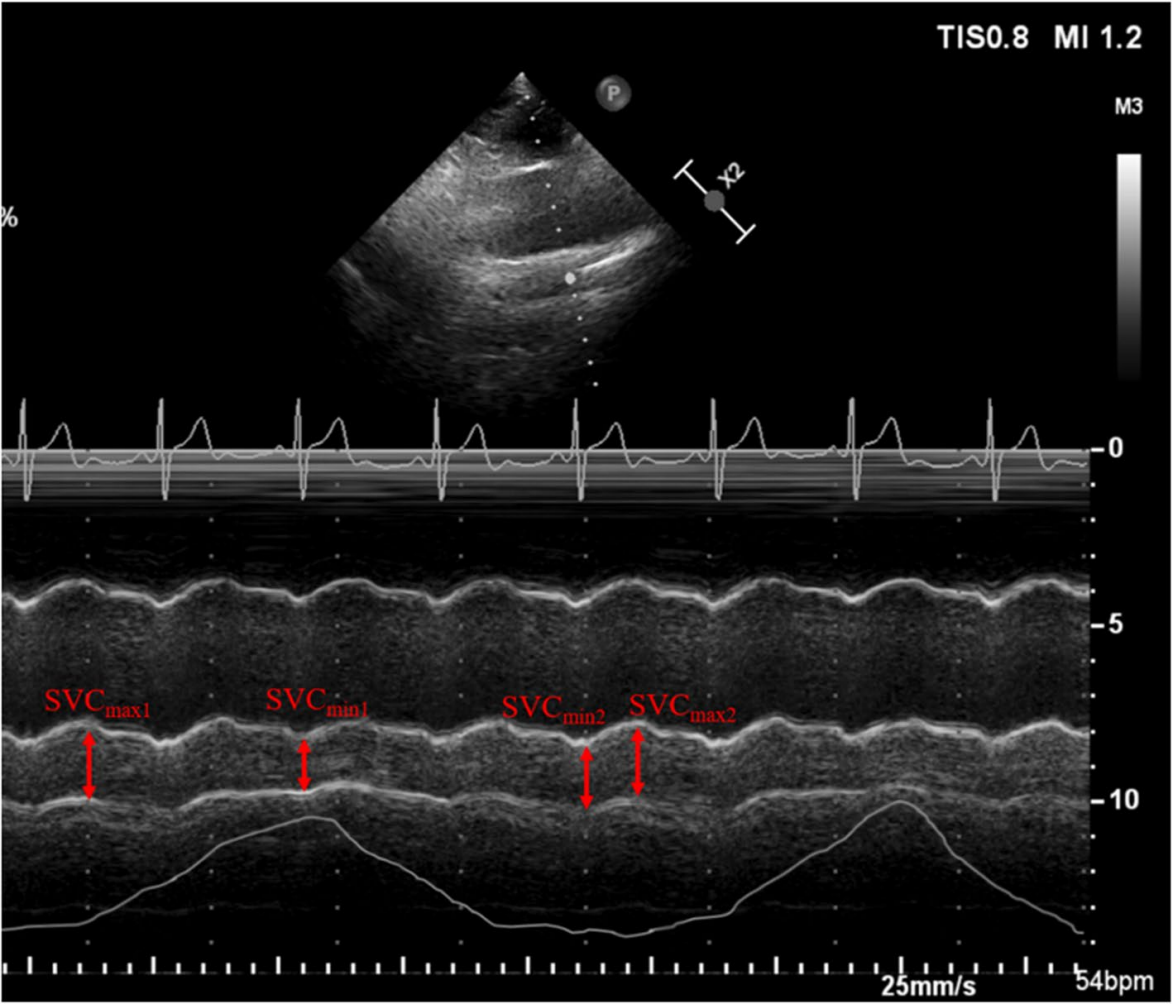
M-mode visualization of the superior vena cava (SVC) measured with transthoracic echocardiography showing the maximal (SVCmax_1_) and minimal (SVCmin_1_) diameters over the respirophasic cycle and maximal (SVCmax_2_) and minimal (SVCmin_2_) diameters over the cardiac cycle
